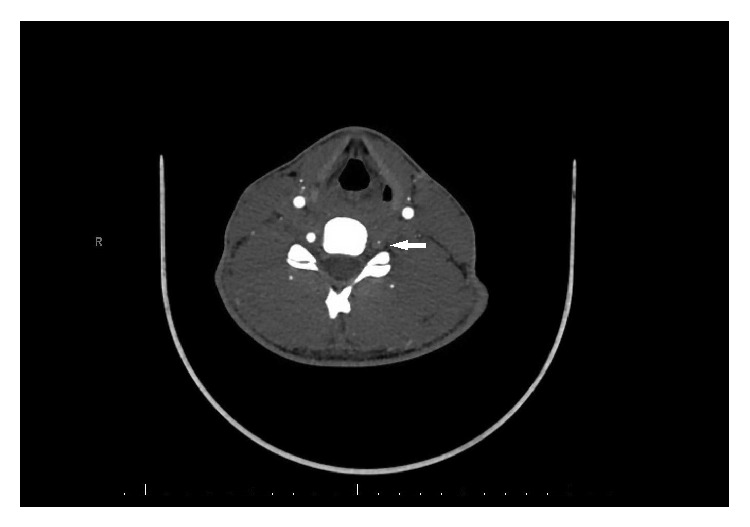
CT Angiogram showing the filling defect in left vertebral artery (marked by white solid arrow).
